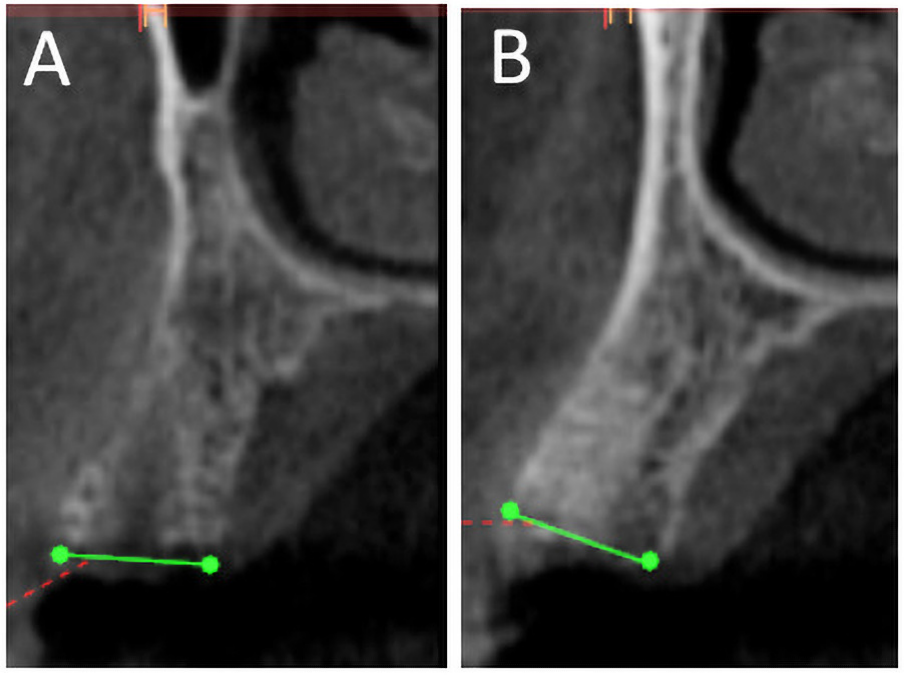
CBCT scan after 6 months for nuOss (A) and Bio-Oss (B) augmented sockets showing more radiopacity for Bio-Oss sites.
